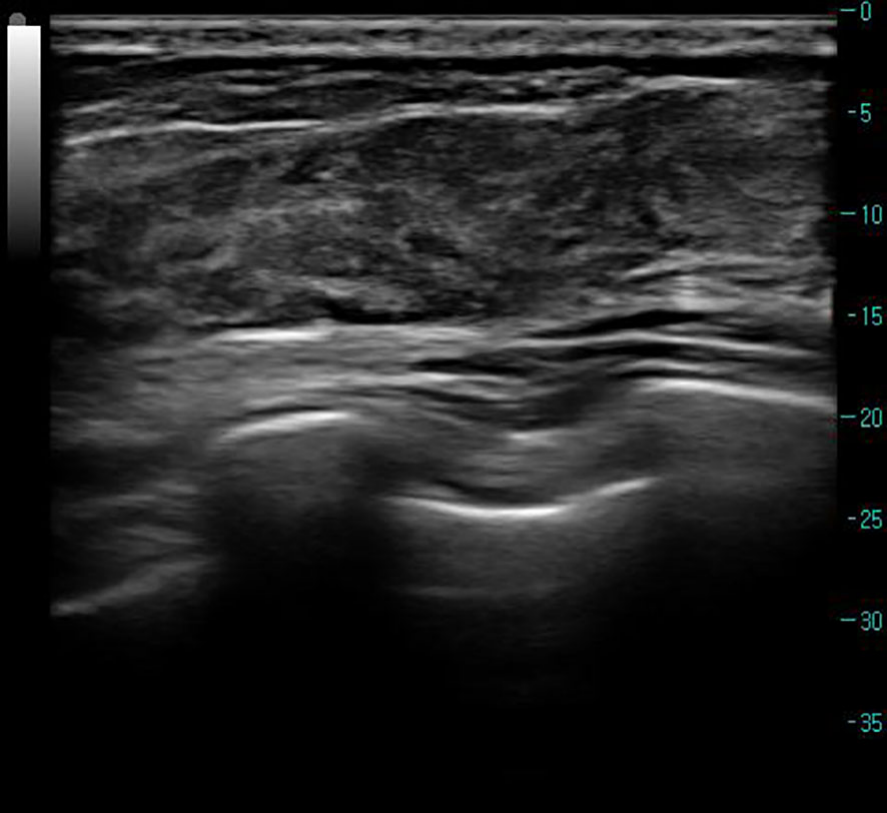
Ultrasound of left breast lesion. Preoperative ultrasound shows a hypoechoic nodule at the junction of the outer lower quadrant of the left breast and the areola, approximately 24 mm × 13 mm in size, with irregular shape, angular edges, uneven internal echo, and no blood flow detected. It is classified as BI-RADS 4a.
